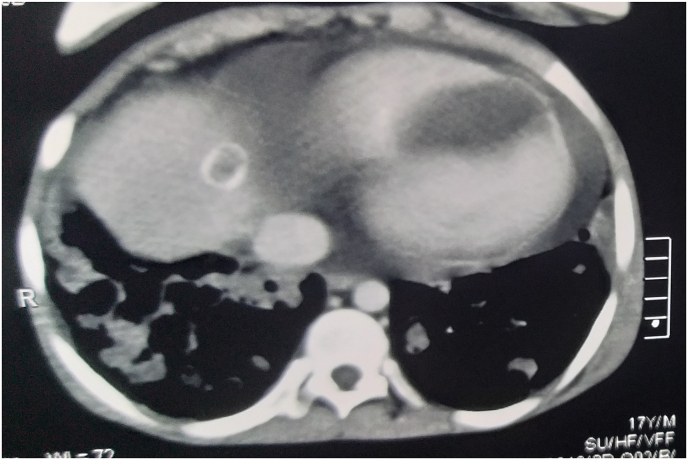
Transverse computed tomography shows hydatid cyst in the distal part of the interventricular septum.
